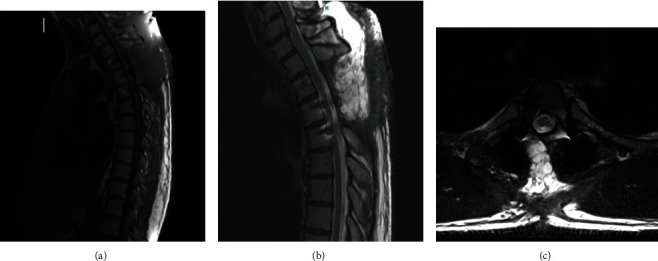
Sagittal, precontrast (a) and postcontrast (b), and axial postcontrast (c) MRI sequences 3 months postoperatively demonstrating maintained surgical decompression and no recurrent cyst formation.
